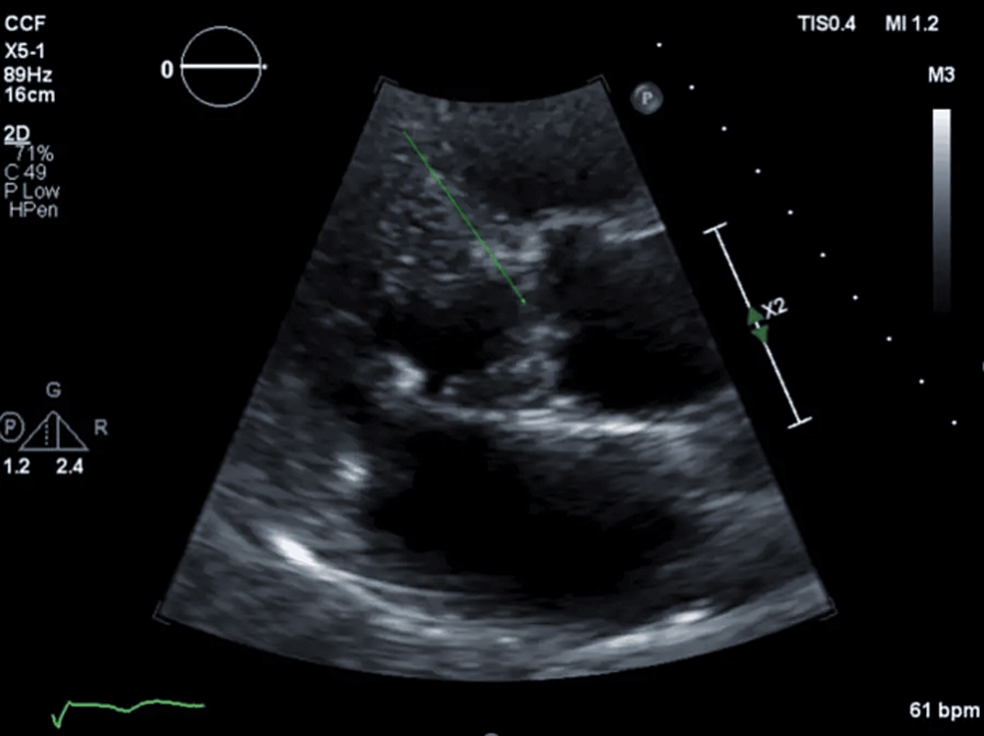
PCI scan Still image from TTE that occurred before PCI. Green arrow shows an intact aortic valve. PCI - percutaneous coronary intervention; TTE - transthoracic echocardiogram
